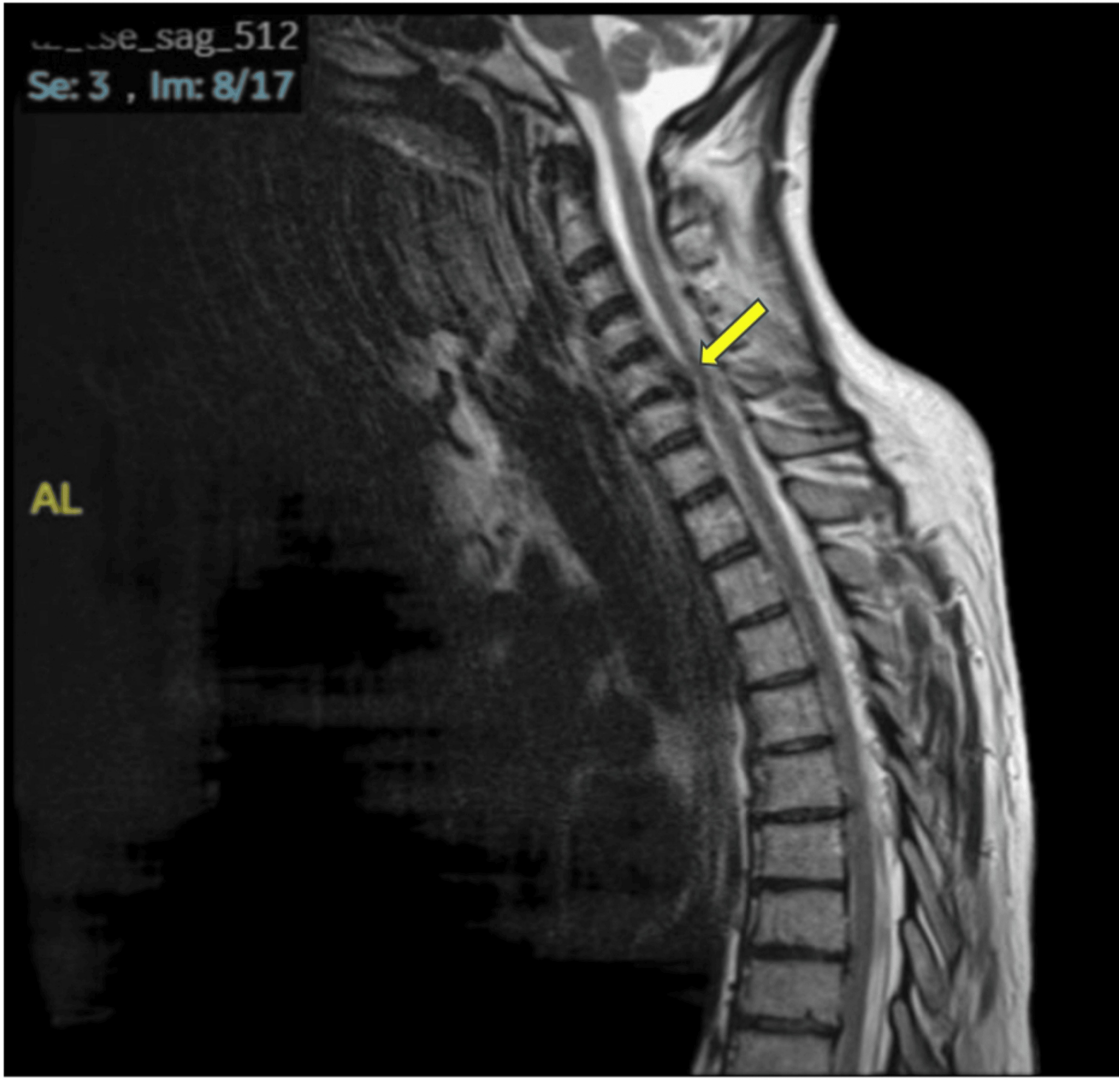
MRI T1 sagittal image of the spine showing a prominent disc bulge at C5/C6 The patient underwent decompression and spinal fusion surgery, but there was no improvement in weakness.
